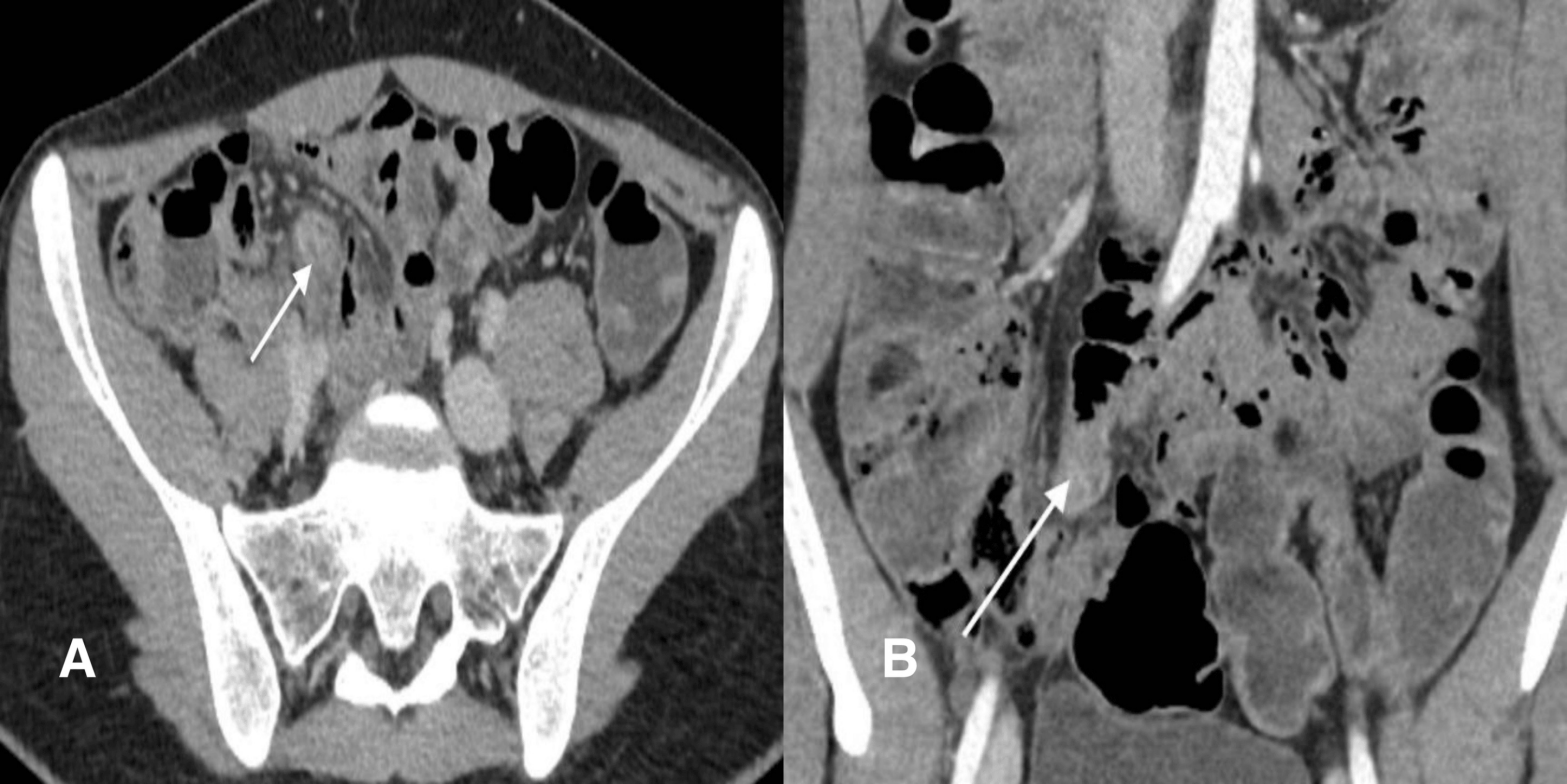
Contrast-enhanced CT abdomen. A: axial CT image showing a blind-ending tubular structure arising from the distal ileum (arrow) with surrounding inflammatory fat stranding, suggestive of Meckel's diverticulitis; B: coronal CT image demonstrating the same blind-ending ileal outpouching (arrow), with adjacent inflammatory changes, supporting the diagnosis of Meckel's diverticulitis.
